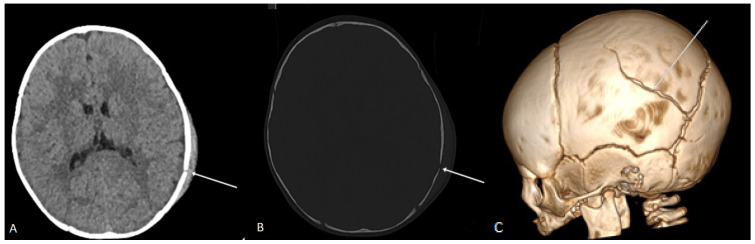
Axial plane CT scan in the soft tissue window (A) and bone window (B) of a 5-month-old child, showing a linear fracture of the left parietal bone with soft tissue edema and a subcutaneous hematoma of the scalp. In the VRT reconstruction, an arrow indicates the communication of the linear fracture of the left parietal bone with the lambdoid suture (C).
